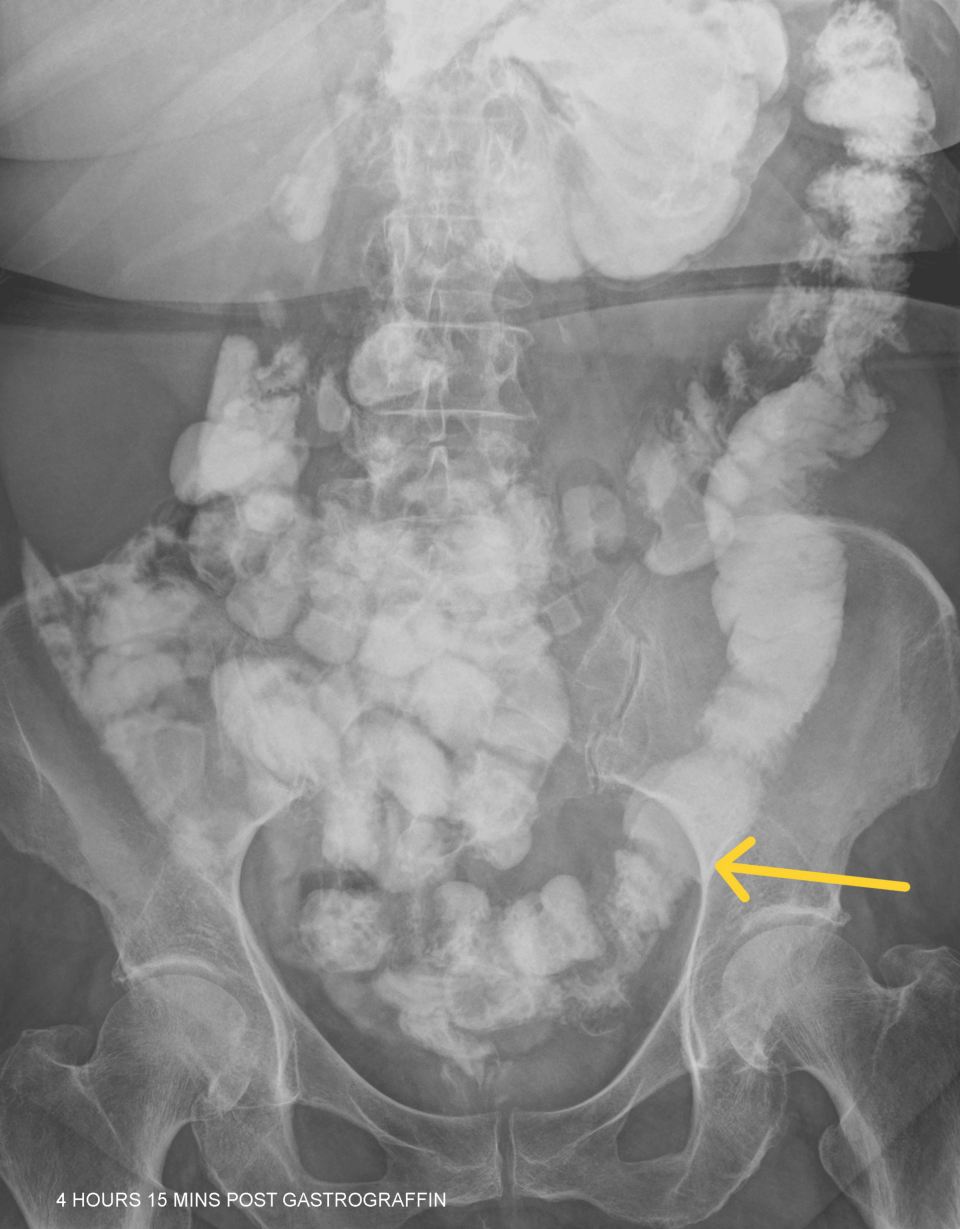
Abdominal X-ray after Gastrografin The yellow arrow demonstrates that the Gastrografin has successfully reached the sigmoid colon.
